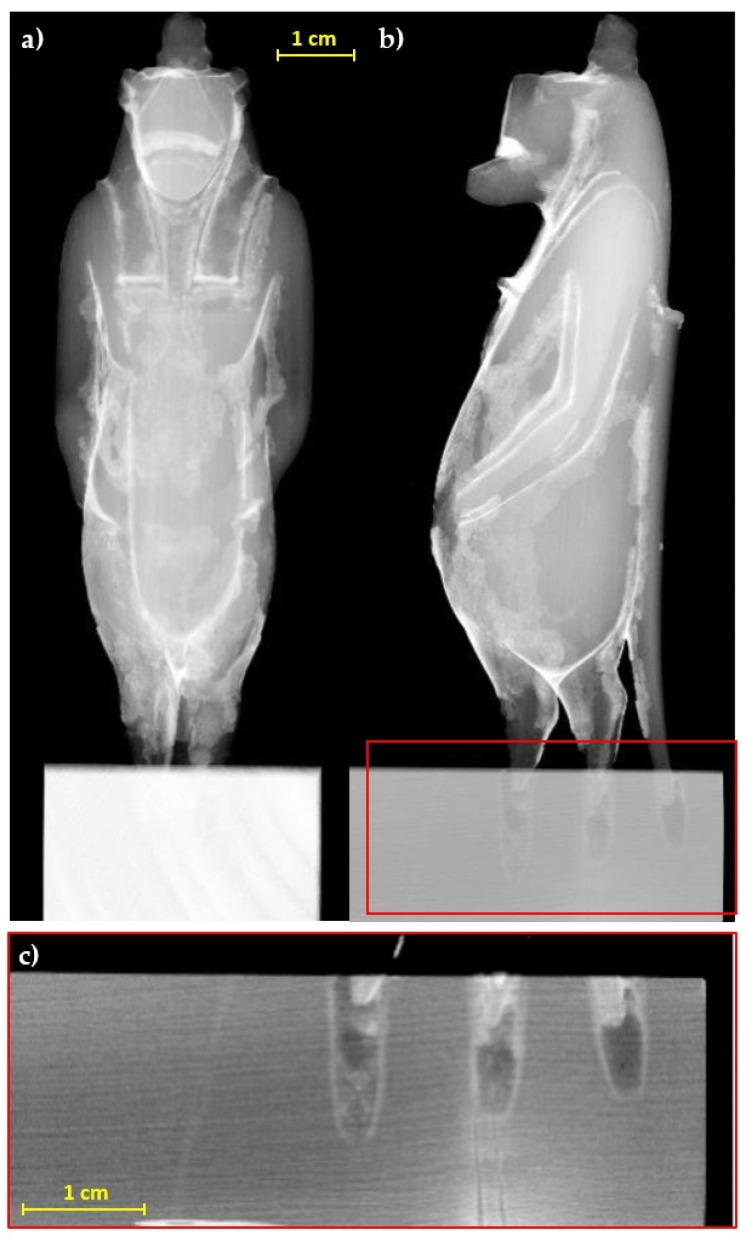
Radiographs of the statuette, (a) frontal and (b) lateral view; (c) detail of the legs and tail insertion in the modern base.
